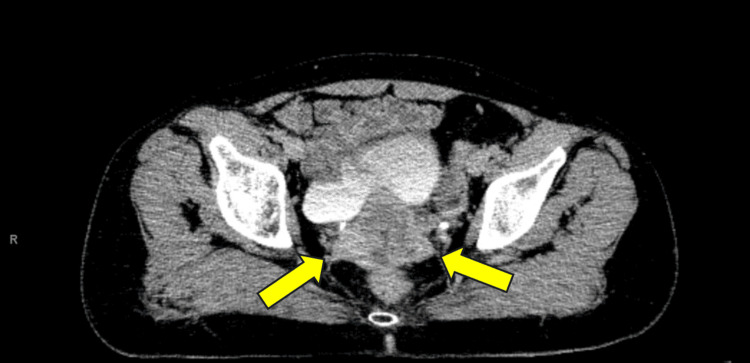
Axial CT image with the yellow arrows pointing to the cervical mass, which measured over 5 cm in size at presentation.
